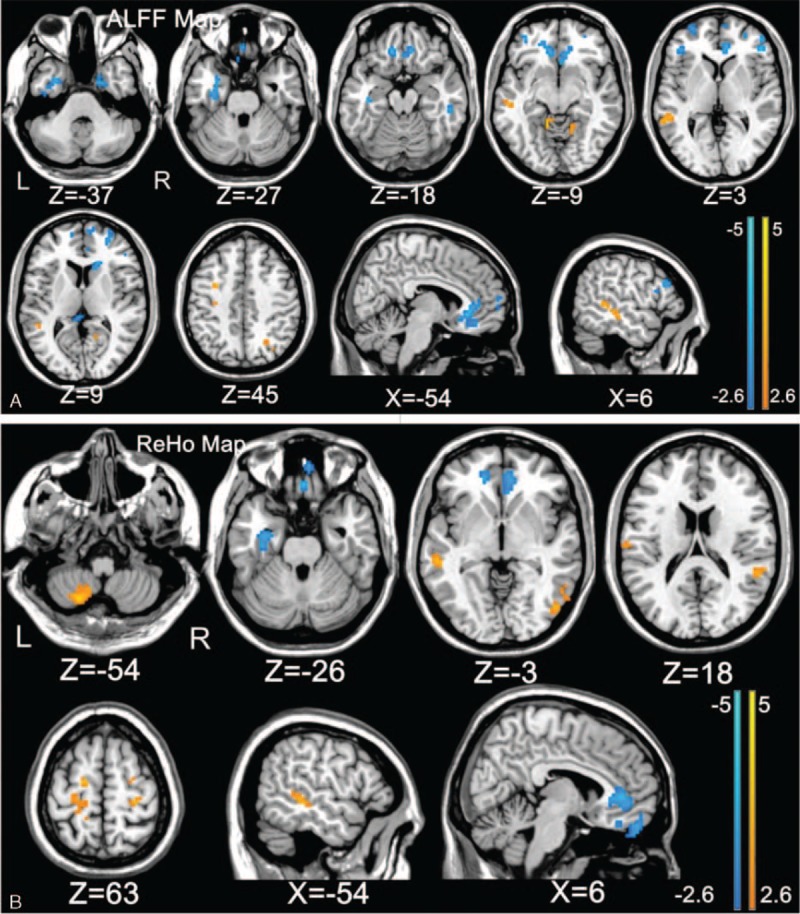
ALFF (A) and ReHo (B) differences between BQD individuals and healthy control subjects (*P* < 0.01, AlphaSim corrected). A, Compared with healthy subjects, patients with BQD showed significantly decreased ALFF in left fusiform, medial prefrontal cortex; right parahippocampal, anterior cingulate cortex; bilateral dorsolateral prefrontal cortex, and increased ALFF in the left precentral, superior temporal gyrus, paracentral lobule; and right calcarine, bilateral lingual. B, Compared with healthy subjects, patients with BQD showed significantly decreased ReHo in the left fusiform, right rectus, and bilateral anterior cingulate cortex and increased ReHo in the left cerebellum posterior lobe, middle temporal gyrus, precentral, paracentral lobule and right superior temporal gyrus, inferior temporal gyrus, inferior occipital gyrus, precentral. For display purposes only, all statistical maps are overlaid on a T1-weighted Montreal Neurological Institute template using MRIcron. ALFF, amplitude of low-frequency fluctuation, BQD = betel quid dependence, ReHo = regional homogeneity.
